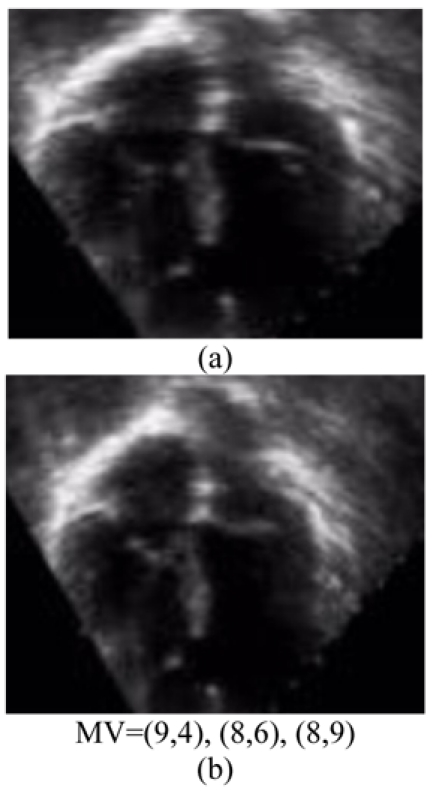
(a) Original ultrasound image and (b) Predicted ultrasound image
